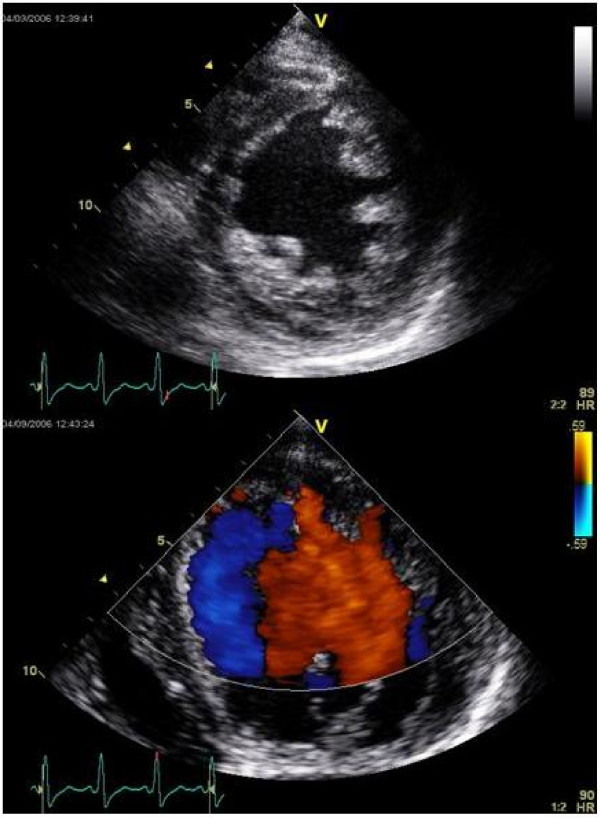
Trans-thoracic echocardiography of our patient, demonstrating prominent trabeculation with colour-flow mapping demonstrating blood flow into the deep intertrabecular recesses in the left ventricle.
